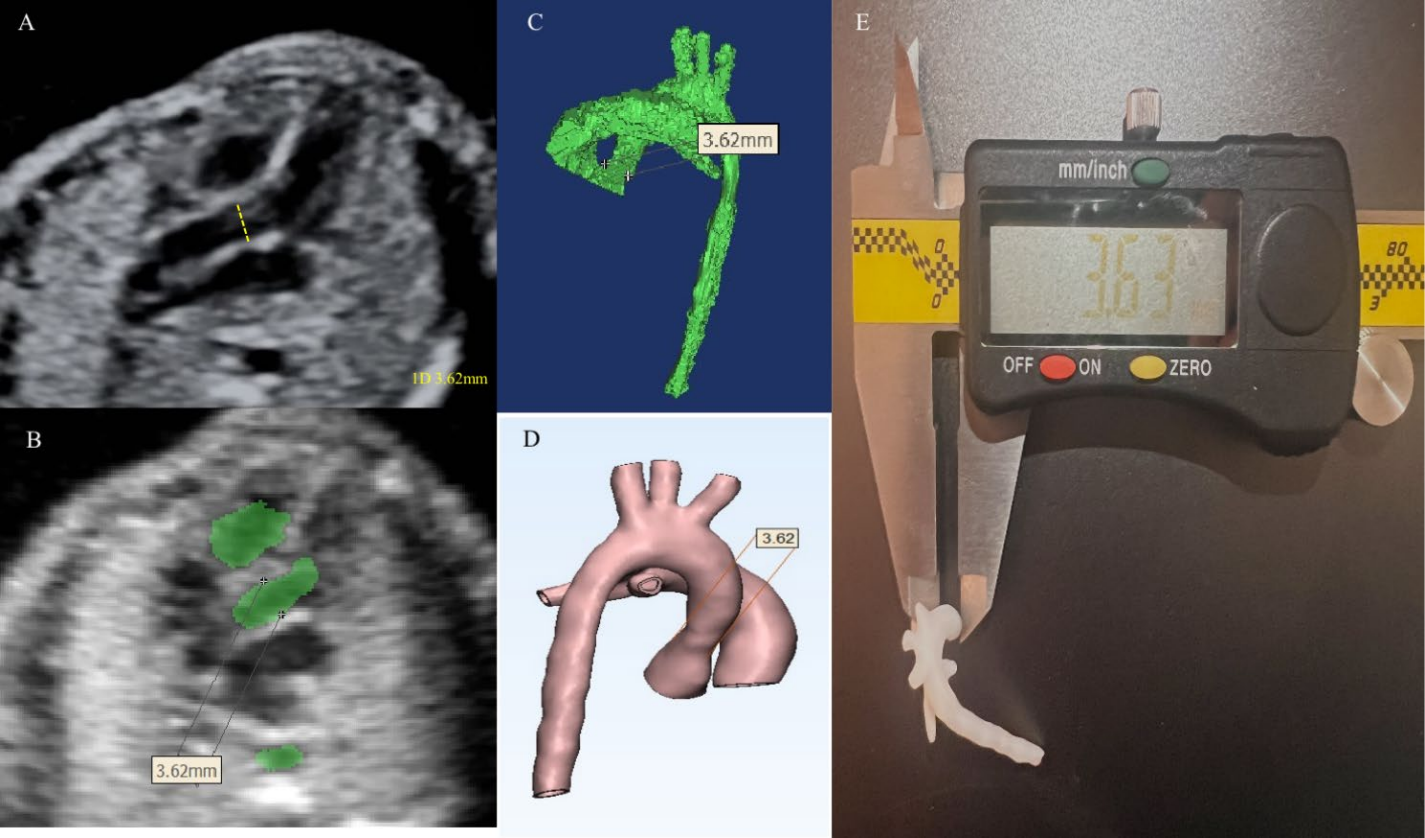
3D model accuracy evaluation. A. Aortic diameter measurement in the echocardiogram. B. STIC original data in the transection view to measure aortic diameter in Mimics. C. 3D object to measure aortic diameter in Mimics. D. 3D digital model to measure aortic diameter in the 3-MATIC. E. Measurement of the aortic diameter in the 3D printed model
